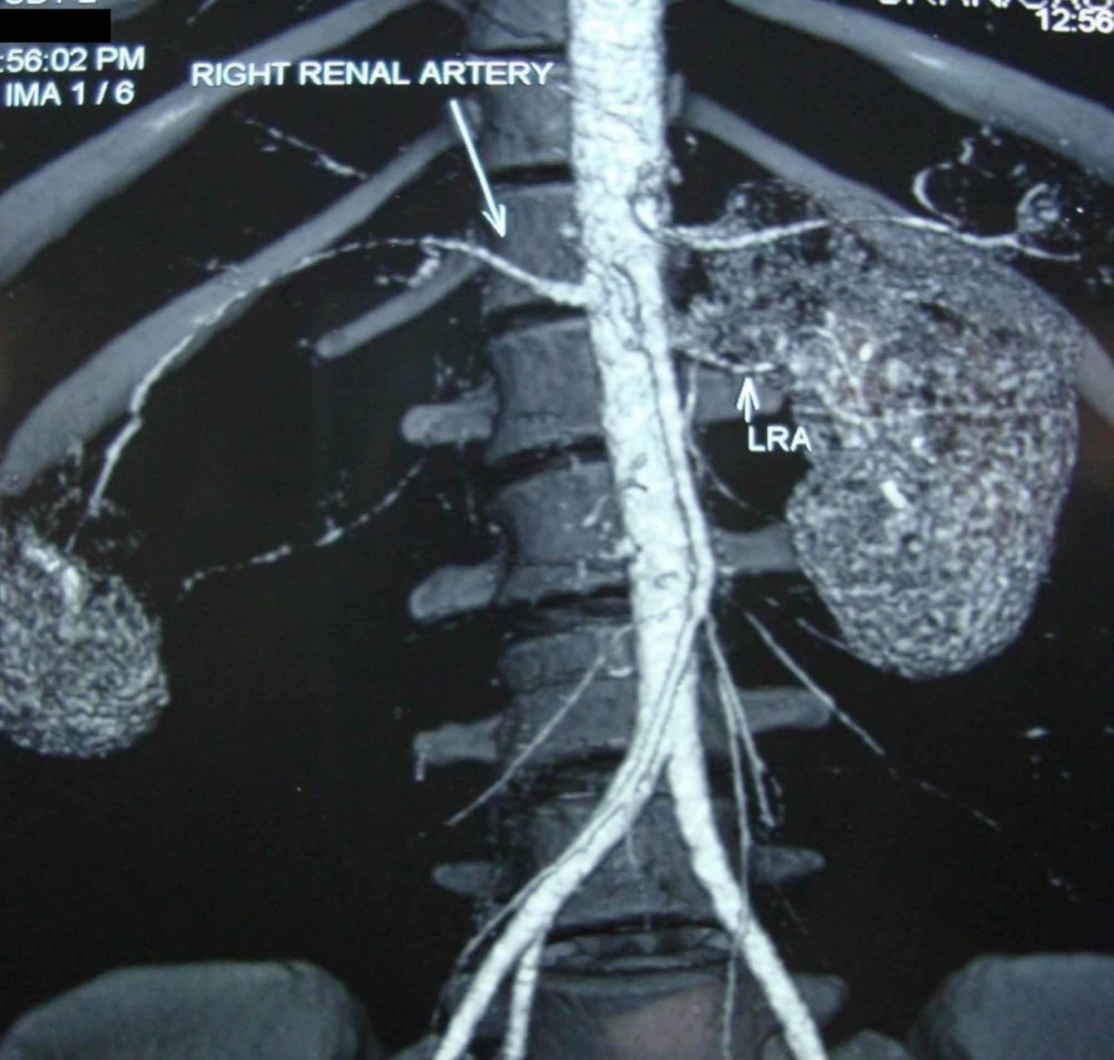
Reconstructed image of the contrast-enhanced computed tomogram depicting the tumour compressing right renal artery (long arrow)
